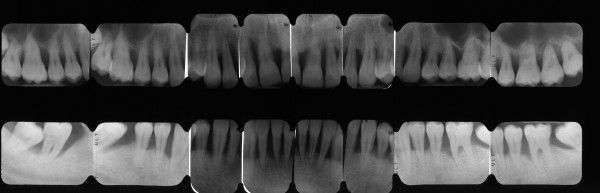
Full-mouth series periapical X-rays. Advanced bone destruction is evident.
